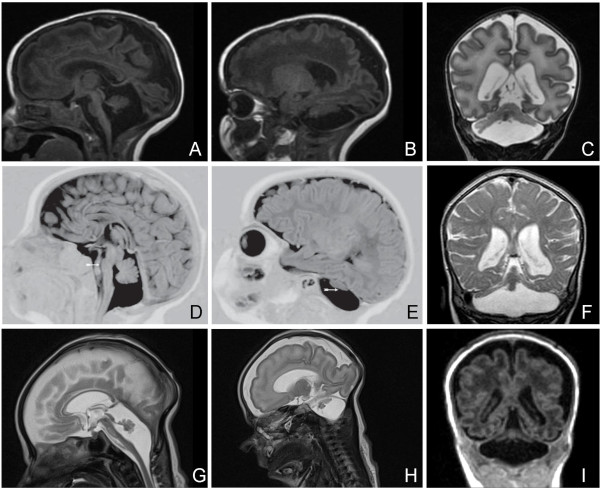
MRI sections of cases with PCH type 1, type 2 and type 4. The images of the PCH1 case were kindly provided by Professor Darin, The Queen Silvia. Children's Hospital, Gothenburg University, Sweden. 1A-C: Images of a 2 wk old neonate with PCH1. 1A: Mid-sagittal section (T1) shows vermal hypoplasia and marked cerebellar hypoplasia. 1B: Lateral sagittal section (T1) shows severe hypoplasia of the cerebellar hemispheres. 1C: Coronal section (T2) shows flattened cerebellar hemispheres which also display some atrophy. The vermis is relatively spared. 1D-E: Images of a 2 months old baby with PCH2. 1D: Mid-sagittal section (T1IR) shows a flat ventral pons and vermal hypoplasia. 1E: Lateral sagittal section (T1IR) shows severely hypoplastic cerebellar hemispheres (arrow) leaving most of the posterior fossa empty. 1F: Coronal section (T2) of a 9 months old infant with PCH2 shows flat cerebellar hemispheres and mild vermal hypoplasia (dragonfly configuration). Cerebral cortical atrophy is also present. 1G-I: Images of a 31+5 weeks neonate with PCH4. 1G: Mid-sagittal section (T2) shows severe vermal hypoplasia and ventral pontine flattening. 1H: Lateral sagittal section (T2) shows severe hypoplasia of the cerebellar hemispheres. Above the tentorium there is an increased distance between the cortical surface and the skull visible, which is probably due to diminished brain growth in utero. 1I: Coronal section (T1) shows extremely small and flattened cerebellar hemispheres and severe vermal hypoplasia. Immaturity of cerebral cortex and enlarged ventricles are also visible.
